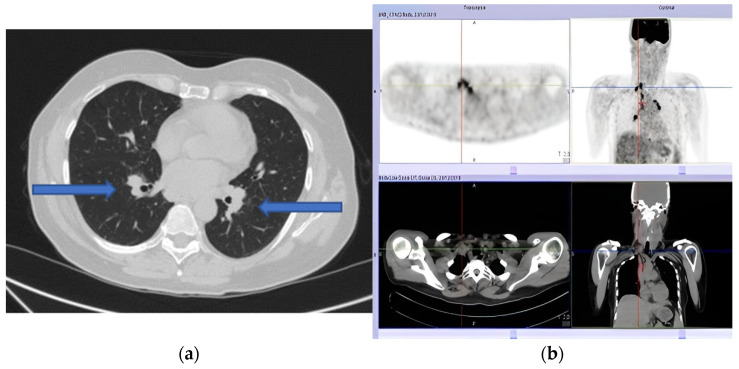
(a) Pulmonary computed tomography (CT) scan showing multiple bilateral lymphadenopathies. (b) Positron emission tomography (PET) showing multiple adenopathies with a high metabolic component.
